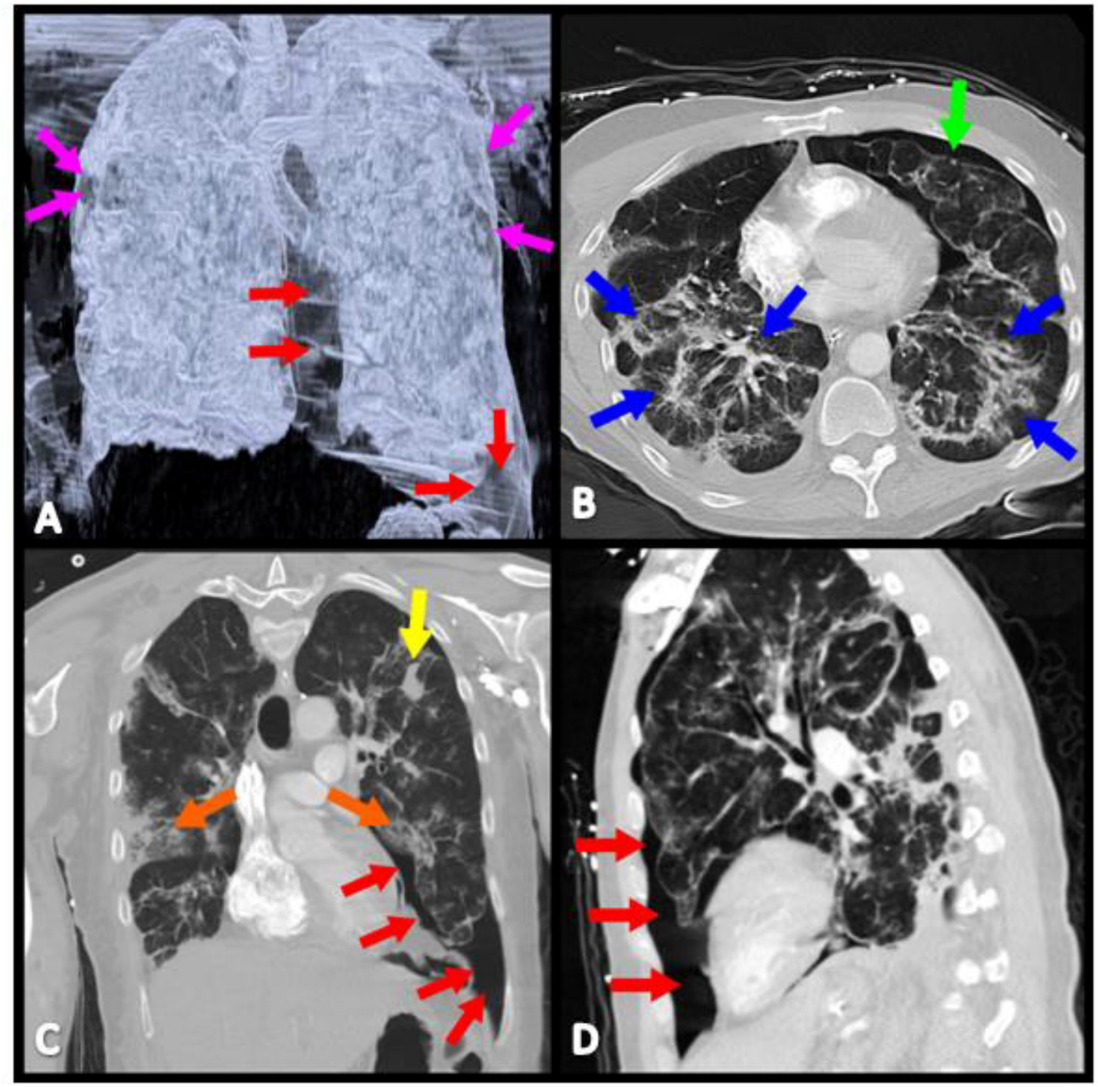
Contrasted CT scan reveals bilateral lung disease, characterized by: (A) Bilateral pneumothoraces (magenta, red, and green arrows). (B) Interstitial, diffuse infiltrates (blue arrows). (C) Isolated areas of consolidation (orange and yellow arrows). (D) Left lung pneumothorax has its largest prominence in the inferior costophrenic sulcus (red arrows).
